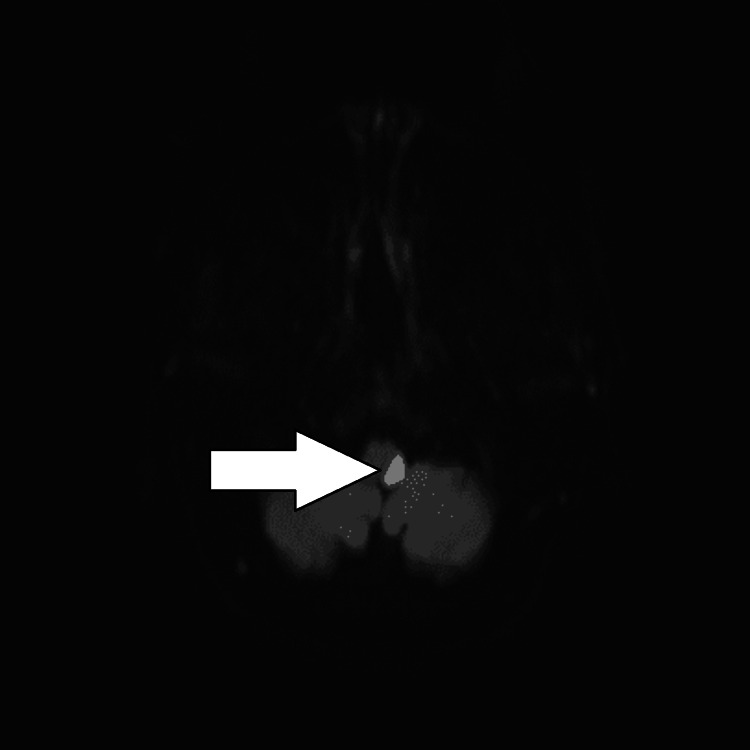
MRI with DWI There is a restricted area of diffusion in the medulla on the left side, which is consistent with an acute medullary infarct on the left side. MRI with DWI remains a key modality in the diagnosis of a medullary infarct. MRI = Magnetic Resonance Imaging; DWI = Diffusion-Weighted Imaging
